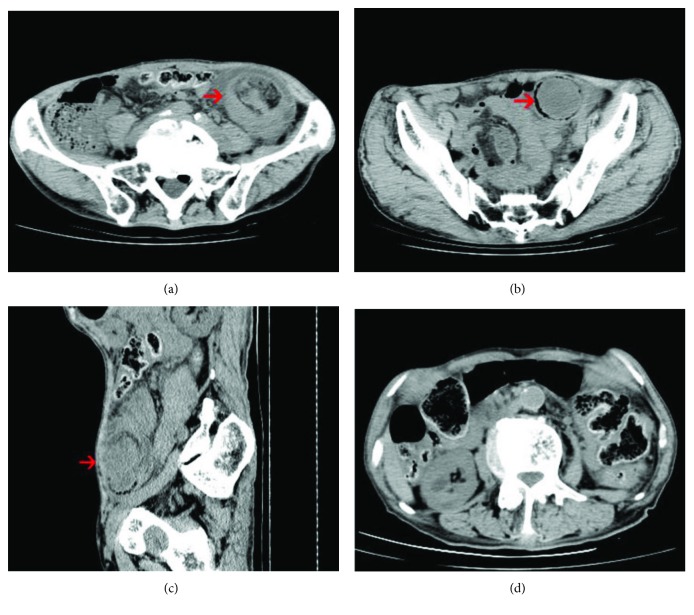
Abdominal CT of Case 1 showing (a) a target sign in the intestinal canal (arrow), (b) colonic schwannoma (arrow), (c) descending colon intussusception (arrow), and (d) intestinal obstruction caused by the schwannoma.
